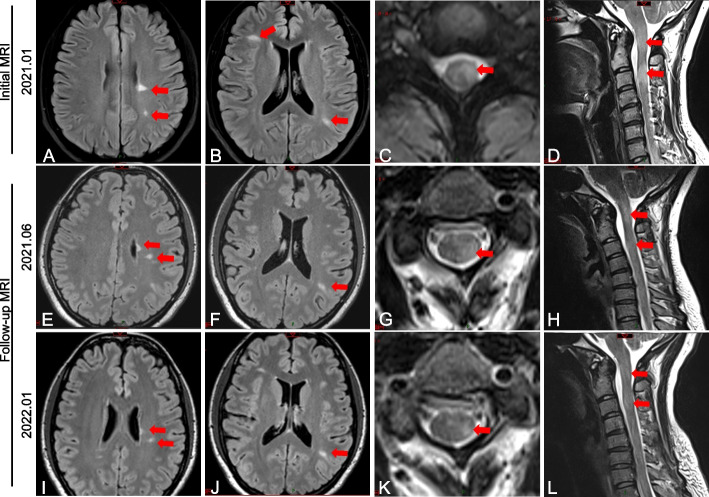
Initial brain and spine MRI of the axial sequence revealing multiple hyperintense lesions at the right anterior part of the upper pons and the left optic nerve (A) and the periventricular and subcortical matter (B) on FLAIR with hyperintense lesions in the left portion part of the cervical spinal cord (C, D). Repeated MRI showed a gradually decrease hyperintensity of periventricular, subcortical matter, and cervical spinal cord lesions after 5 months (E–H) and 1 year (I-L) after teriflunomide treatment
